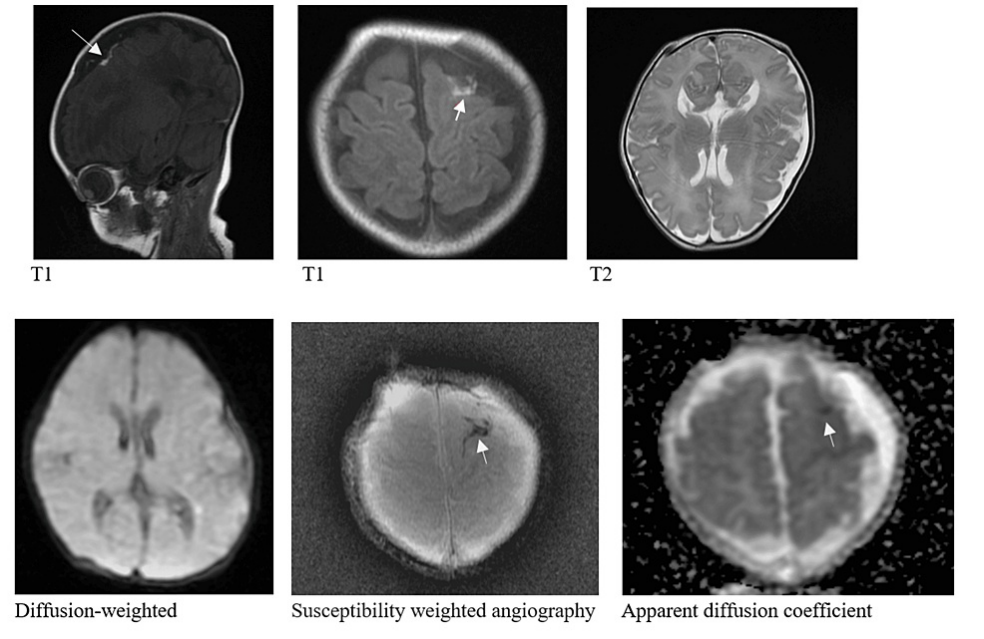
Brain magnetic resonance images at six days of life showed a small, left-sided subdural hemorrhage (arrows)
